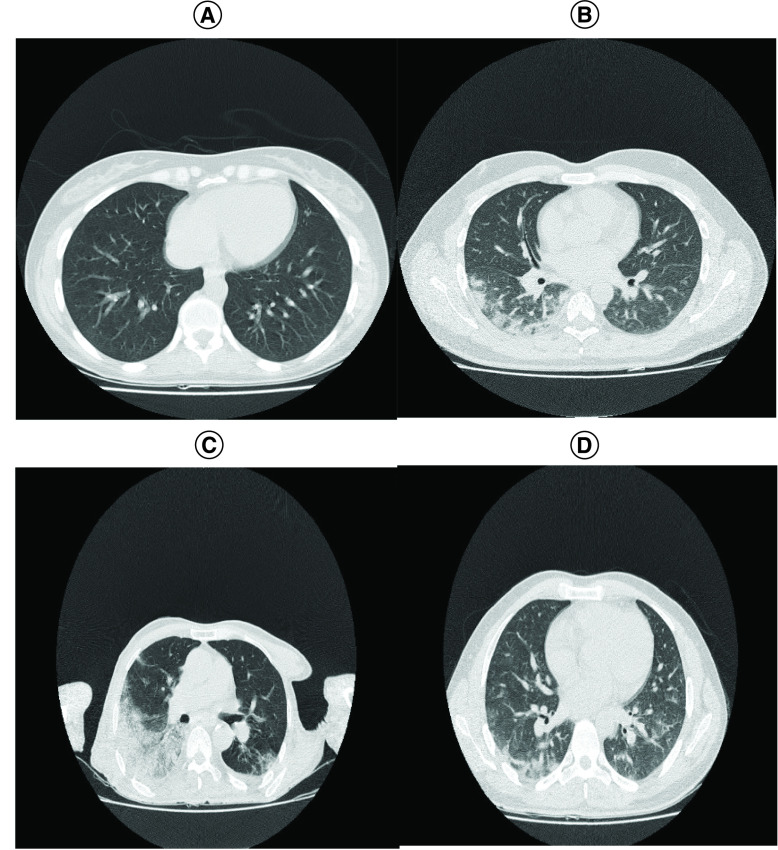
Representative computed tomography images of patients with coronavirus disease-2019. (A) Female, 27 years old (outpatient) with fever and dyspnea. Axial ccmputed tomography (CT) image showed normal lung markings. (B) Female, 35 years old (inpatient with alive outcome) with fever and dyspnea. Axial CT image showed small bilateral areas of ground-glass opacities. (C) Male, 74 years old (elderly) with fever, cough and dyspnea. Axial CT image shows larger ground-glass opacities in the right lung. (D) Male, 31 years old (nonelderly) with fever and cough. Axial CT image shows ground-glass opacities in the bilateral lungs.
